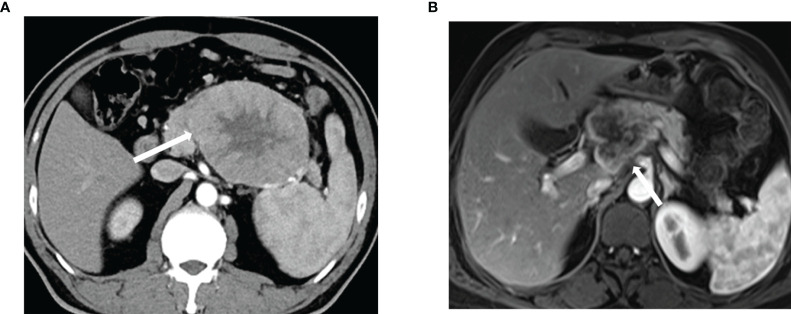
Biphasic contrast enhanced computed tomography and magnetic resonance imaging of ACC (A) Venous enhancement is heterogeneous. (B) There is uneven enhancement of lesions in the portal arterial phase. ACC, acinar cell carcinoma.
